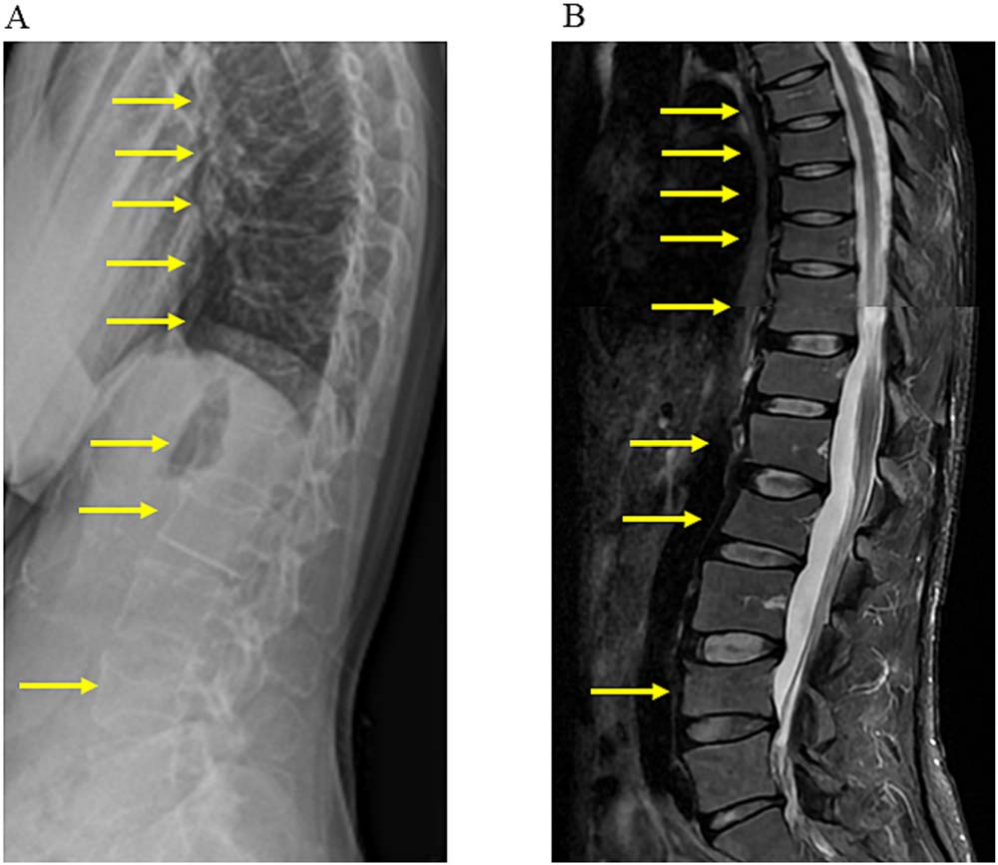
Lumbar spine imaging after treatment. (A) Lateral radiograph of the vertebrae. (B) Lateral STIR magnetic resonance imaging of the vertebrae. Arrows indicate vertebral bodies fractured before treatment. No progression of vertebral collapse or new vertebral fractures was observed.
